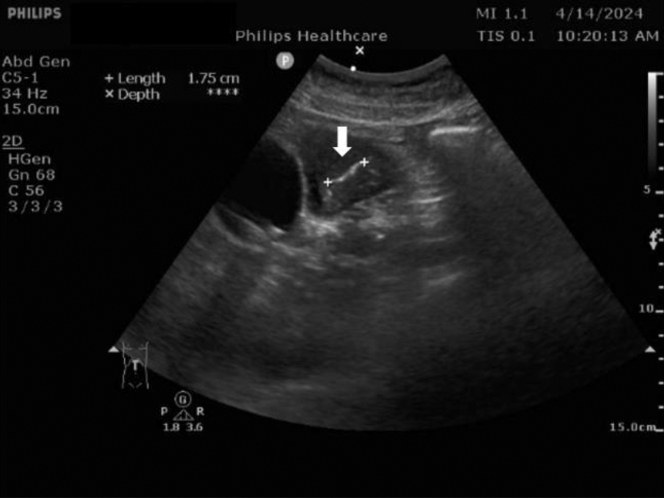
Bedside point-of-care ultrasonography showing a hyperechoic structure with acoustic shadow (arrowhead) over the stomach.
